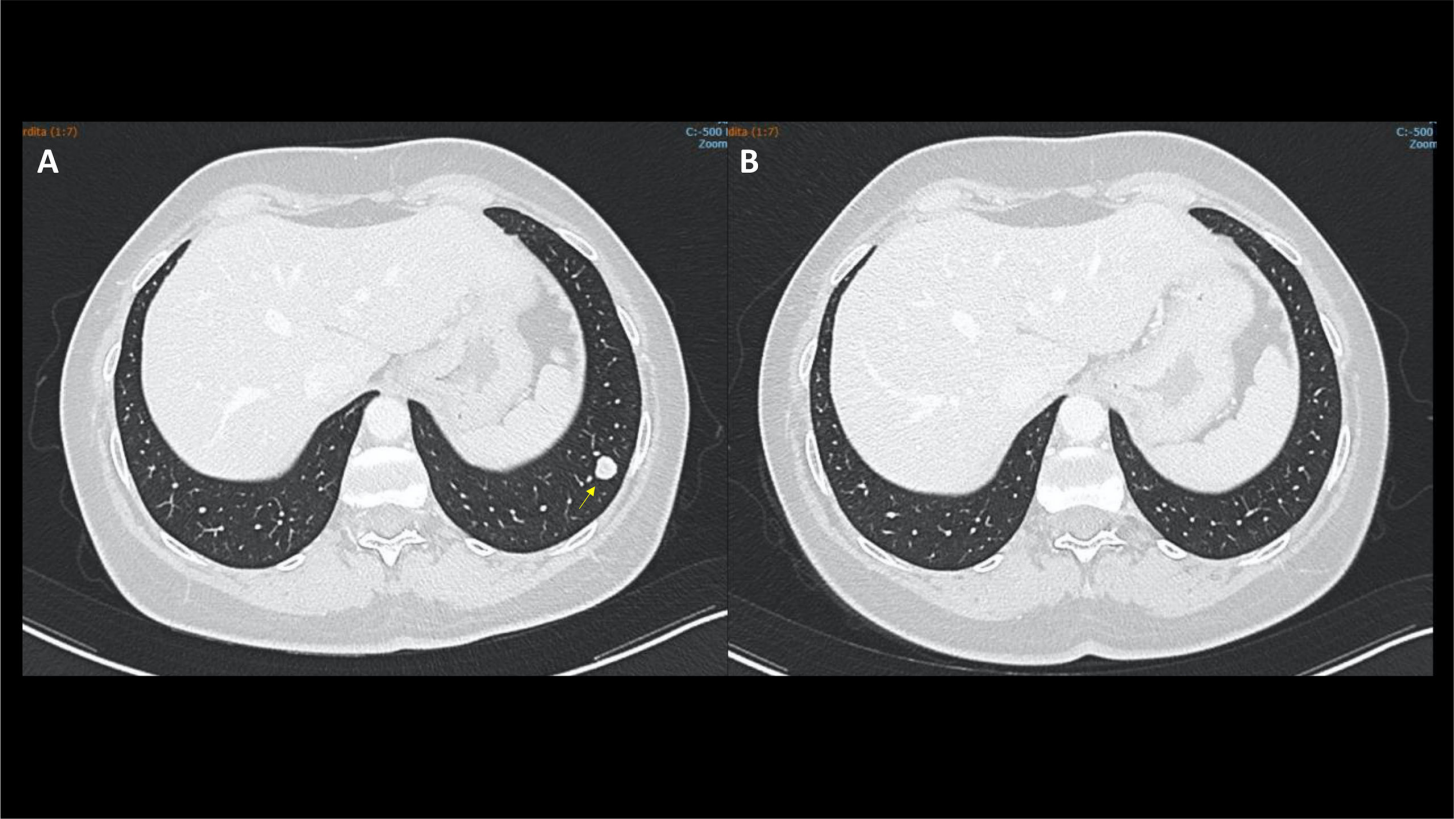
Case 1, CT scan showing a significant shrinking in the left lung lower lobe (arrows) from December 2021 (A) to May 2022 (B).
